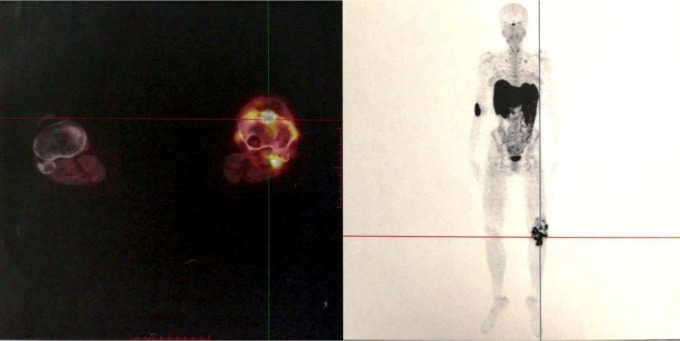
^68^Ga-DOTATATE-PET/CT: multiple lesions with high levels of somatostatin receptor in the soft tissue around the left knee joint and the proximal left tibia.
